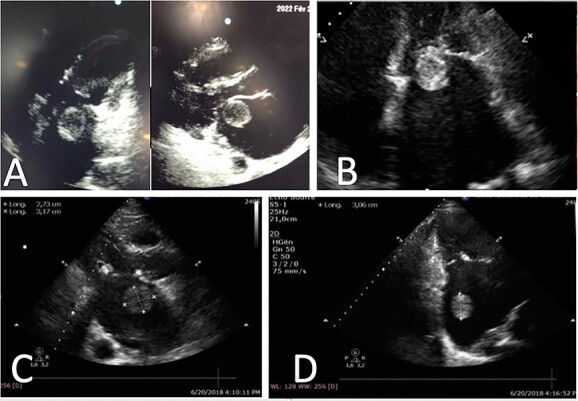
Representative echocardiography images of thrombus ball, (A) TTE views of a thrombus ball blocking the mitral valve (case 1), (B) Apical four chamber view on TTE showing a huge left atrial thrombus ball in contact with mitral valve (case 2), (C) and (D) Left atrial round thrombus on TTE (case 3).
